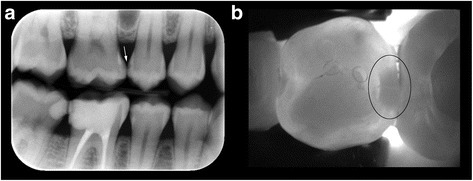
a PSP-Bitewing radiograph showing proximal caries lesions in both the second premolar (scale 1) and first molar (scale 3) (arrow), (b) NILT image showing the carious lesion only for the second premolar (scale 1) and without any caries lesion (scale 5) for the first molar teeth
